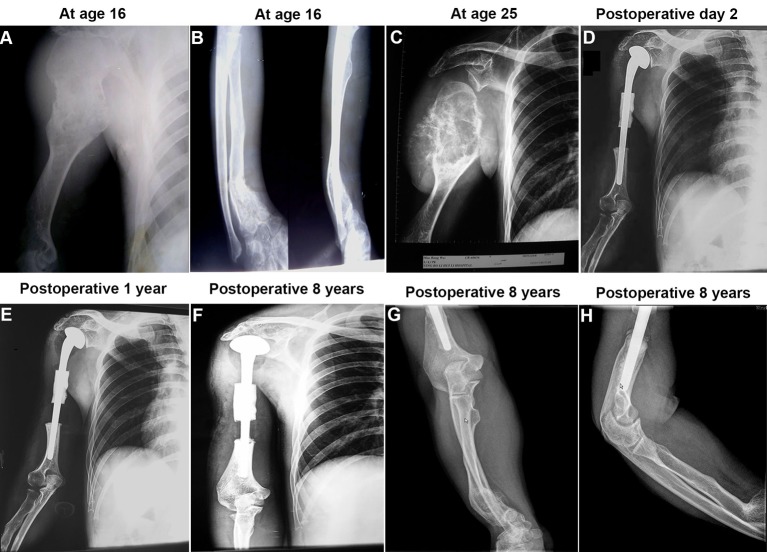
Radiographic evaluation of the surgical treatment at pre- and postoperative stages. (A and B) Radiograph showing cartilaginous lesions or enchondromas predominantly localized in the upper part of the humerus (A) and the lower part of the radius (B) of the 16 years old boy affected with Ollier disease. (C) A huge tumor of the proximal humerus with massive cortical destruction and soft tissue extension was noticed at age 25 of the patient. (D) The radiograph on the postoperative day 2 (anteroposterior view). The prosthesis was well in place after the surgery. (E) The radiograph on 1 year after the surgery (anteroposterior view). The prosthesis was still well in place. (F) The radiograph on 8 years after the surgery (anteroposterior view). The prosthesis remained well in place. (G and H) The radiographs of the ulna and radius of the limb were also taken at age 33 of the patient (8 years after the surgery). There were no signs for malignant transformation in those lesions.
